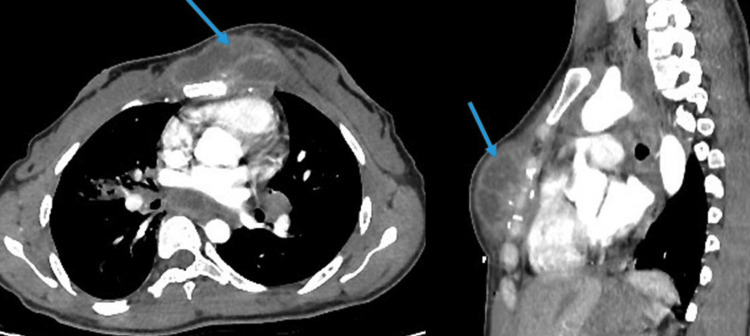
Axial (A) and sagittal (B) sections of the contrast-enhanced CT scan Images showing a left-sided presternal mass (blue arrow) with calcification
